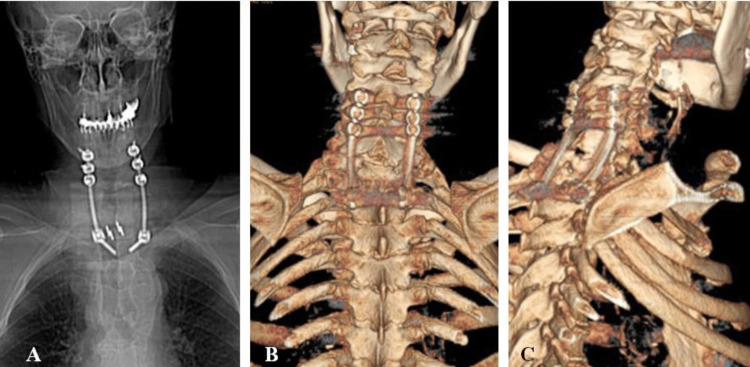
Postoperative crevicothoracic CT scan and 3D reconstruction (Case 1) (A) posterior long segment stabilization and implanted cage T1-T2; (B, C) Postoperative CT 3D reconstruction (front and profile) – good reconstruction and stabilization 3D: three-dimensional
